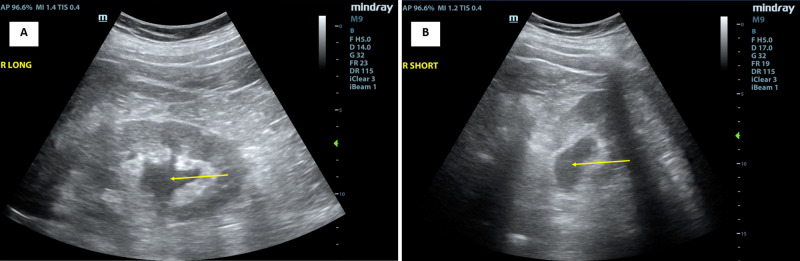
Right-sided renal ultrasound images Right-sided renal ultrasound images in the long-axis view (A) and the short-axis view (B) demonstrate moderate hydronephrosis (marked by arrows).
